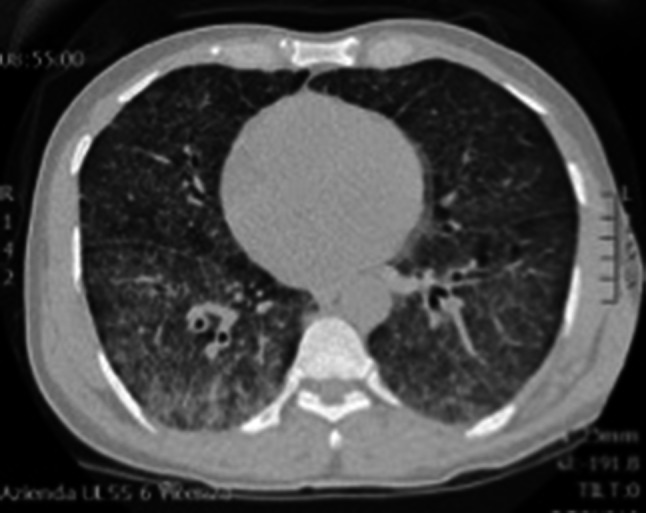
Chest CT scan showing ground-glass appearance and interstitial thickening due to interstitial pneumonia caused by the underlying generalized CMV infection
